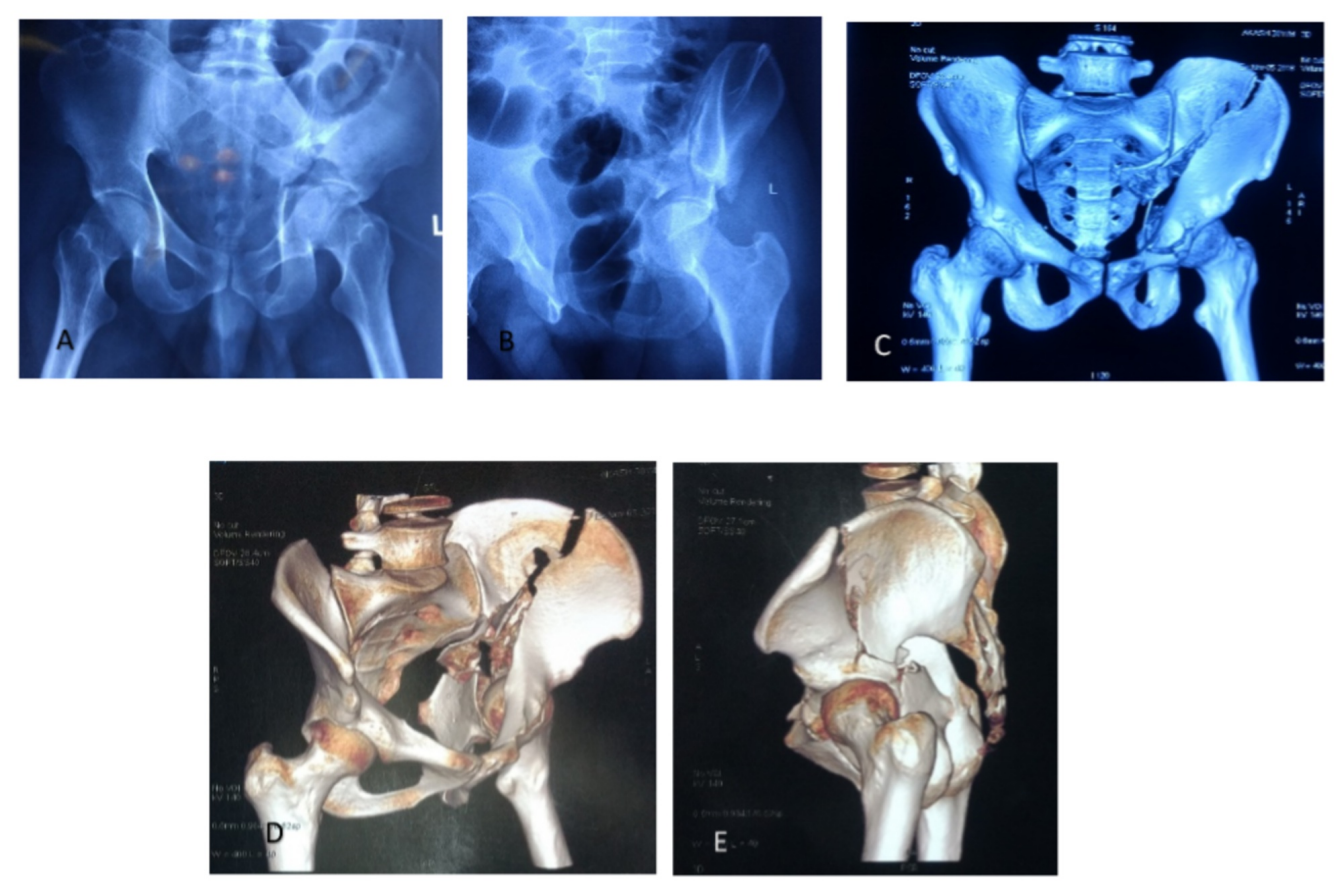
Preoperative x-rays and 3D-CT pelvis showing both-column fracture of left acetabulum: A) Anterior posterior view radiograph of pelvis; B) obturator view radiograph of pelvis; C,D,E) 3D-CT pelvis.
